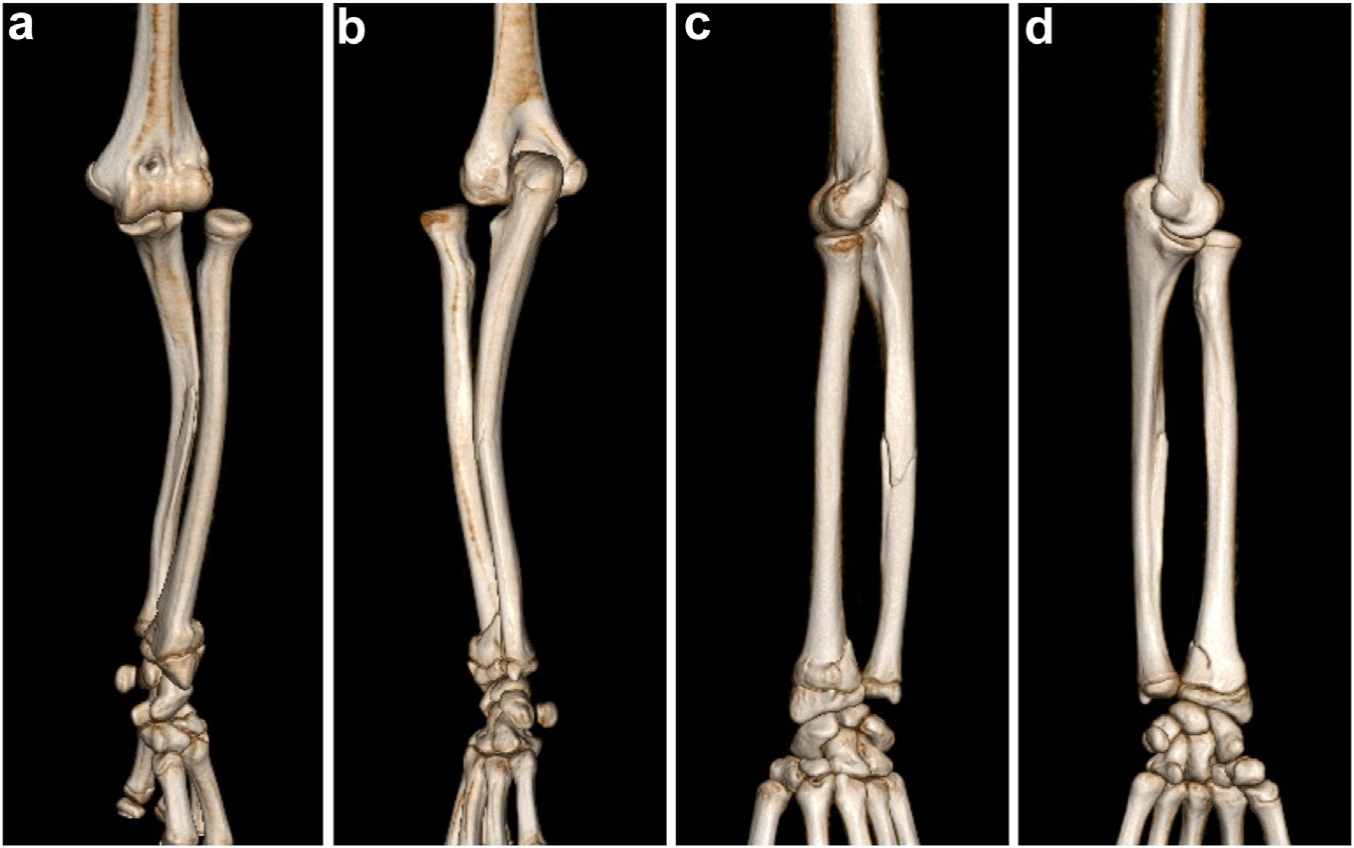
Three-dimensional CT images showing lateral dislocation of the radial head in the anterior and posterior view (a, b) and slightly anterior displacement in the lateral view (c, d). *CT*, computed tomography.
